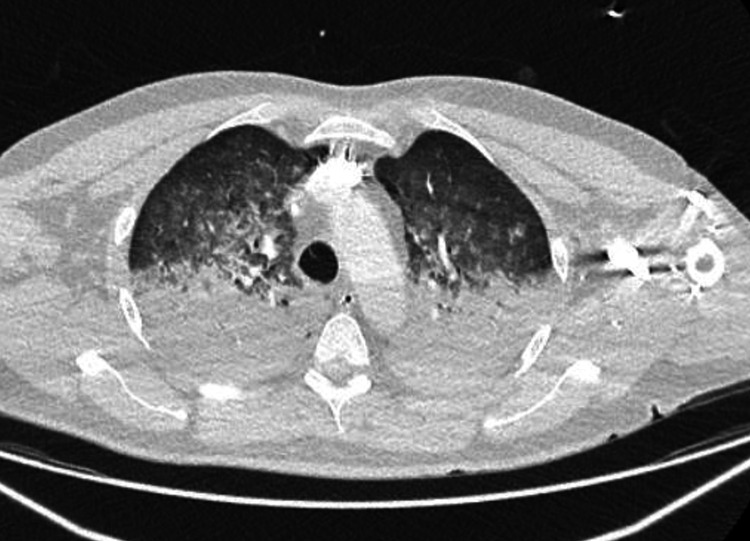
A CT scan of the chest before ICU admission showing bilateral lung extensive consolidations
